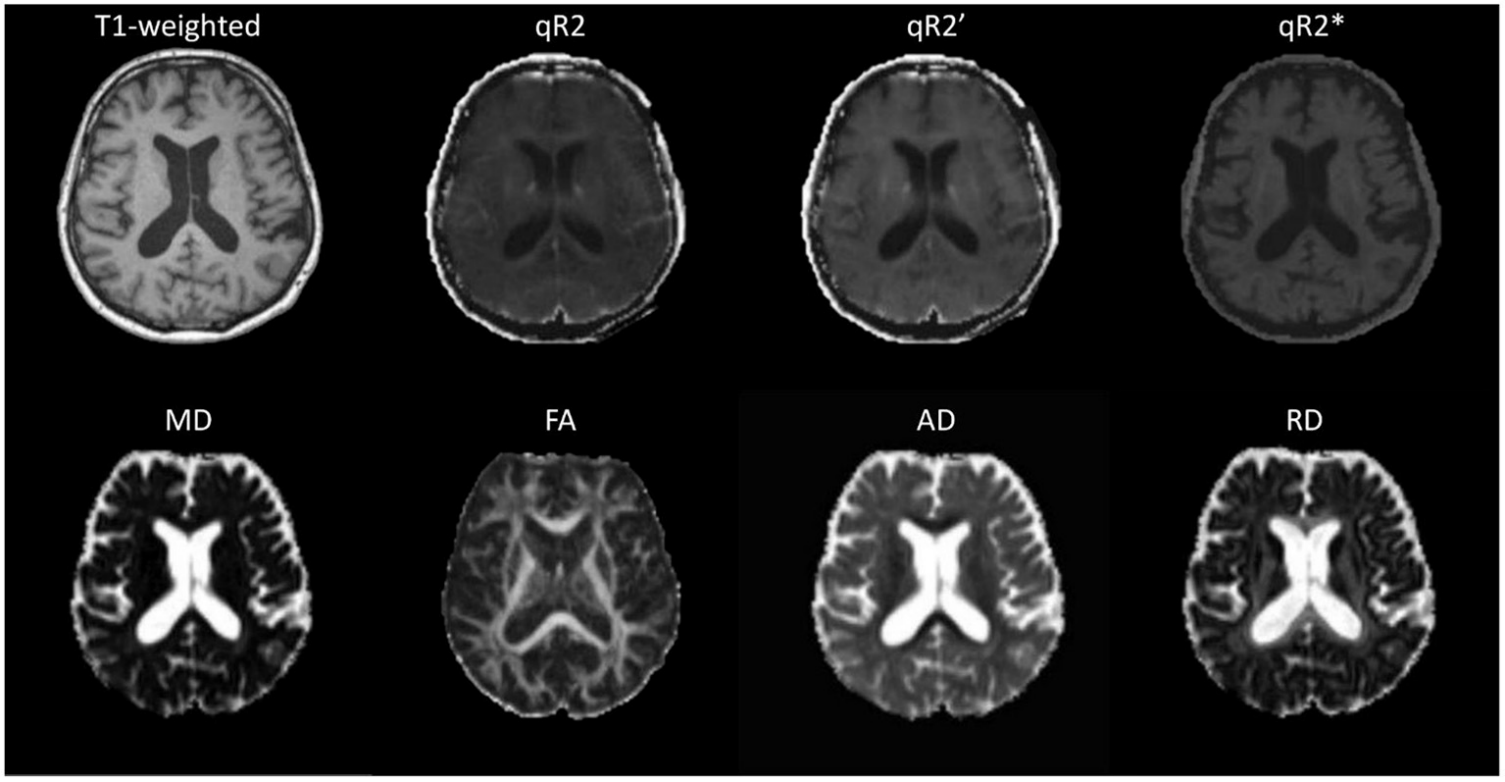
Selected slice from a multi-modal MRI dataset of a patient with Parkinson's disease.
